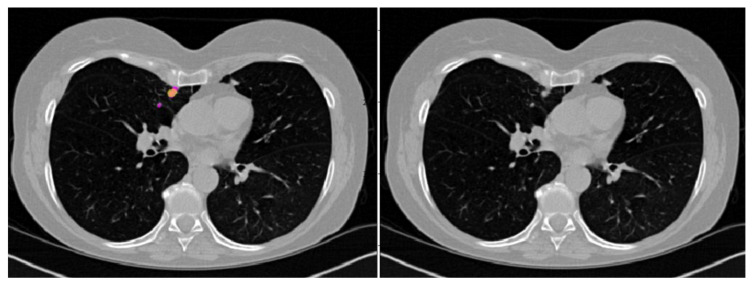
Example of a nodule close to pleura in the right lung correctly predicted by the CADe and of a small nodule, near to the previous one, missed by the CADe. Left panel: axial CT slice with prediction (yellow) and/or mask (pink); right panel: original CT image.
